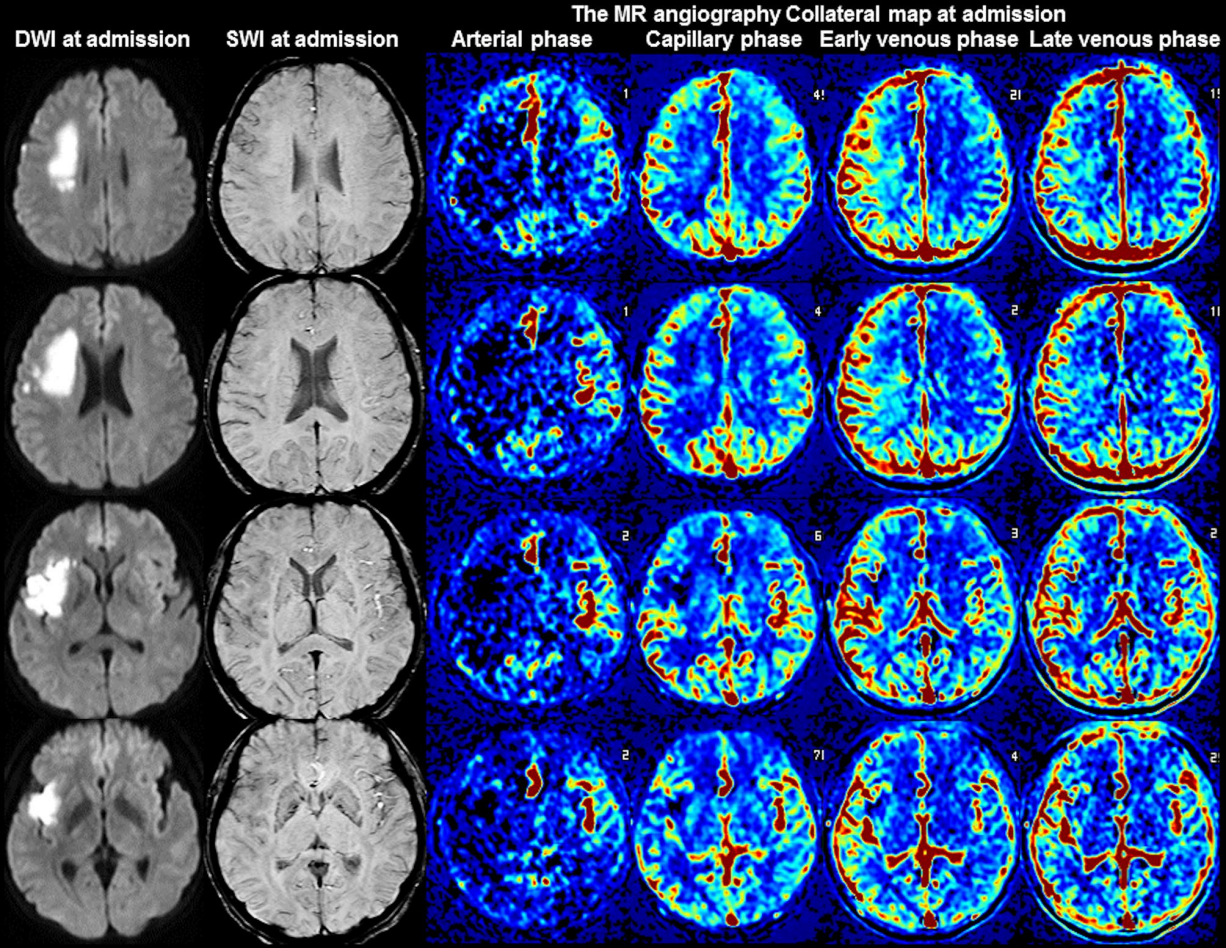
Images of a 55-year-old woman with occlusion of the right proximal internal carotid artery. The premorbid modified Rankin scale (mRS) score of this patient was 0, and the National Institutes of Health Stroke Scale score at admission was 4. The diffusion-weighted imaging (DWI), susceptibility-weighted imaging (SWI), and multiphase MR angiography collateral map at 4 h 45 min after symptom onset are shown. DWI shows acute infarct signals in the right frontal and temporal lobes, and SWI shows mildly prominent cortical veins in the right middle cerebral artery territory, representing intermediate collateral status. The MR angiography collateral map shows collateral-perfusion delay of more than one-half of the right middle cerebral artery territory in the capillary phase and small collateral-perfusion delay around the insula in the early venous phase (MR acute ischemic stroke collateral score of 3: intermediate to good collateral perfusion defined as capillary-perfusion delay of more than one-half of the ischemic MCA territory in the capillary phase and no or small delay in the early venous phase). The patient underwent bypass surgery and recovered, as shown by the 90-day mRS score of 2.
